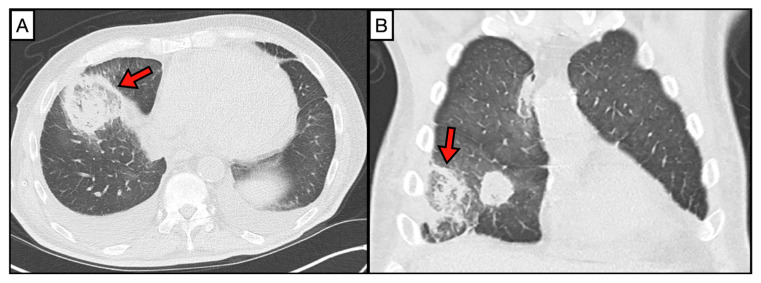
Reverse halo sign (red arrow) in (A) axial and (B) coronal chest CT images from a patient with pulmonary mucormycosis caused by *Rhizopus arrhizus*.
